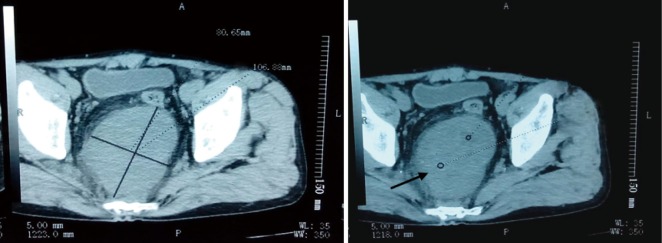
Abdominal CT: two pre-operation images of the abdomen. From the images, we can observe a large quasi-circular tumor, which is about 10.7 cm × 8.1 cm in the sacro-anterior. The tumor is intensified asymmetrically and its boundary is clear. The surrounding fat gap of the tumor is clear and no lymph node was found. CT, computerized tomography.
